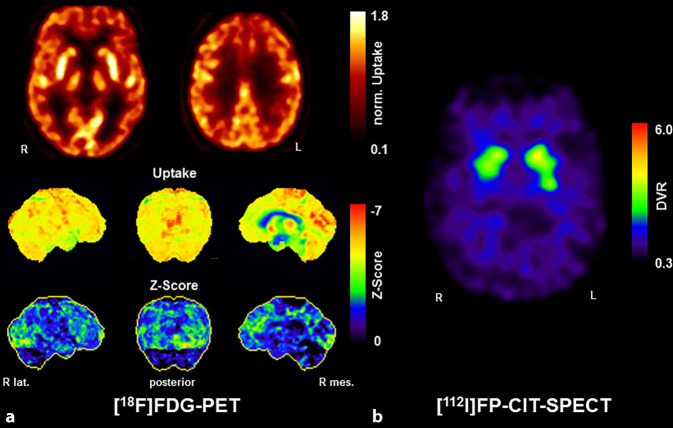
Sehr vielversprechende Studienergebnisse liegen nun auch zum neu definierten MCI mit Lewy-Körpern vor (sog. MCI-LK): Sowohl für die [^123^I]FP-CIT-SPECT als auch die [^123^I]MIBG-Szintigraphie wird eine hohe Spezifität (je 88 %) und moderate Sensitivität (66 % bzw. 59 %) für die Trennung zwischen wahrscheinlichem MCI-LK und MCI infolge AD beschrieben [72, 73]. Ähnliche präliminäre Ergebnisse fanden sich für die [^18^F]FDG-PET (AUC-ROC ca. 0,95 [74]). Sehr interessant sind auch erste Ergebnisse einer kombinierten [^123^I]FP-CIT-SPECT- und Amyloid-PET-Studie bei MCI-LK. Diese ergab, dass eine Beteiligung des nigrostriatalen Systems in der DAT-SPECT („D+“) zwar deutlich häufiger als ein Aβ-positiver Befund ist (A− D+, 38 %; A+ D+, 27 %; A− D− 27 %; A+ D− 9 %), aber nur der A+-Status mit schlechterer Kognition korrelierte (ebenso mit höherem Alter und APOE-E4-Status). Die nigrostriatale Integrität korrelierte hingegen mit der Motorik, Fluktuationen und RBD [75]. Inwieweit diese Beobachtungen zur individuellen Risikostratifizierung herangezogen werden können, werden entsprechende Follow-up-Studien zeigen.
